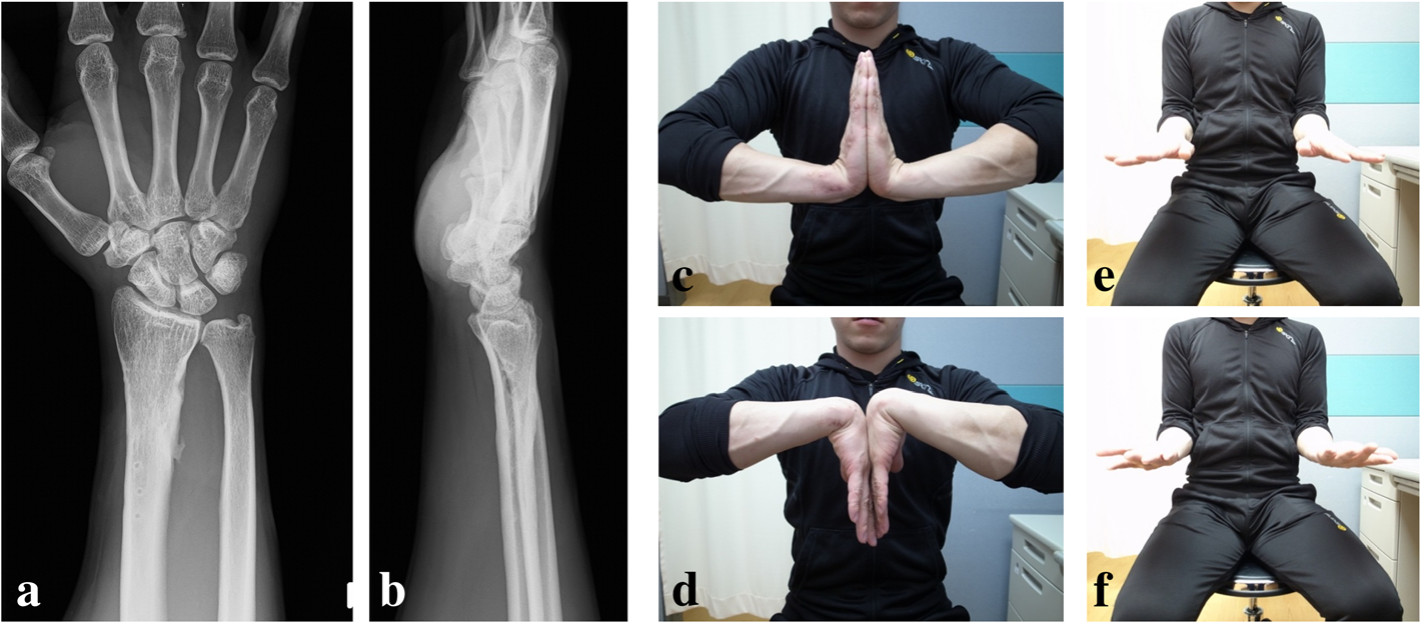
Plain radiograph and clinical photograph at the final follow-up 15 months postoperatively. (a) Anteroposterior view and (b) lateral view of the right wrist. (c) Dorsal flexion of the wrist, (d) palmar flexion of the wrist, (e) pronation of the forearm, and (f) supination of the forearm
